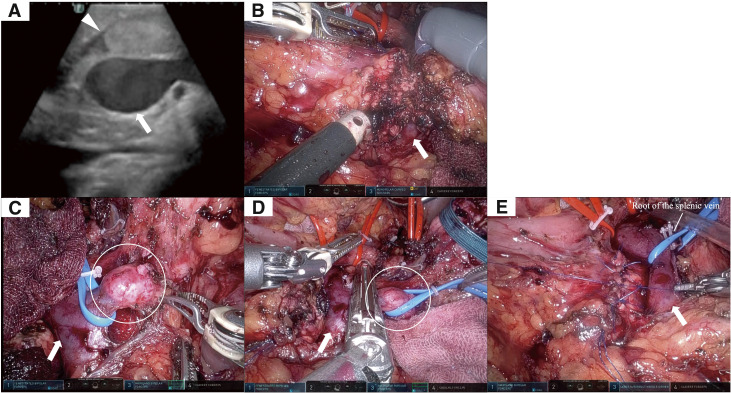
Intraoperative findings. Arrowhead: tumor; arrows: SMV. (A) Intraoperative ultrasonography revealed tumor extension into the main pancreatic duct just above the SMV. (B) Robotic surgery enabled sharp transection of the pancreas at the right edge of the SMV using monopolar curved scissors. (C) The IMV directly merged with the SMV, and a tumor thrombus (circle) was observed in the IMV. The tumor thrombus did not extend to the junction with the SMV. (D) The IMV was transected at its confluence with the SMV using a linear stapler (circle: tumor thrombus). (E) The pancreatic stump was precisely closed with sutures by the multi-joint function of robotic surgery. IMV, inferior mesenteric vein; SMV, superior mesenteric vein
